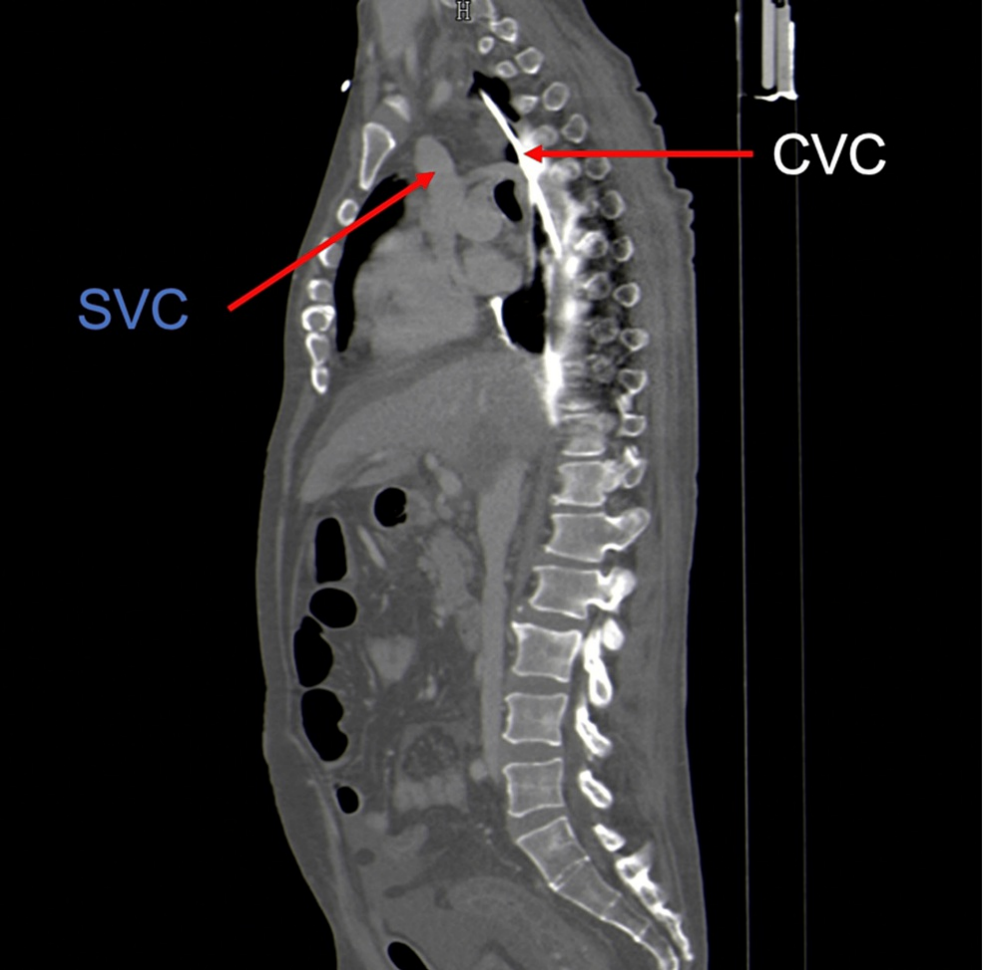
CTA scan showing extravascular CVC path along the posterior border of the SVC (sagittal view) CTA, computed tomography angiography; CVC, central venous catheter; SVC, superior vena cava
